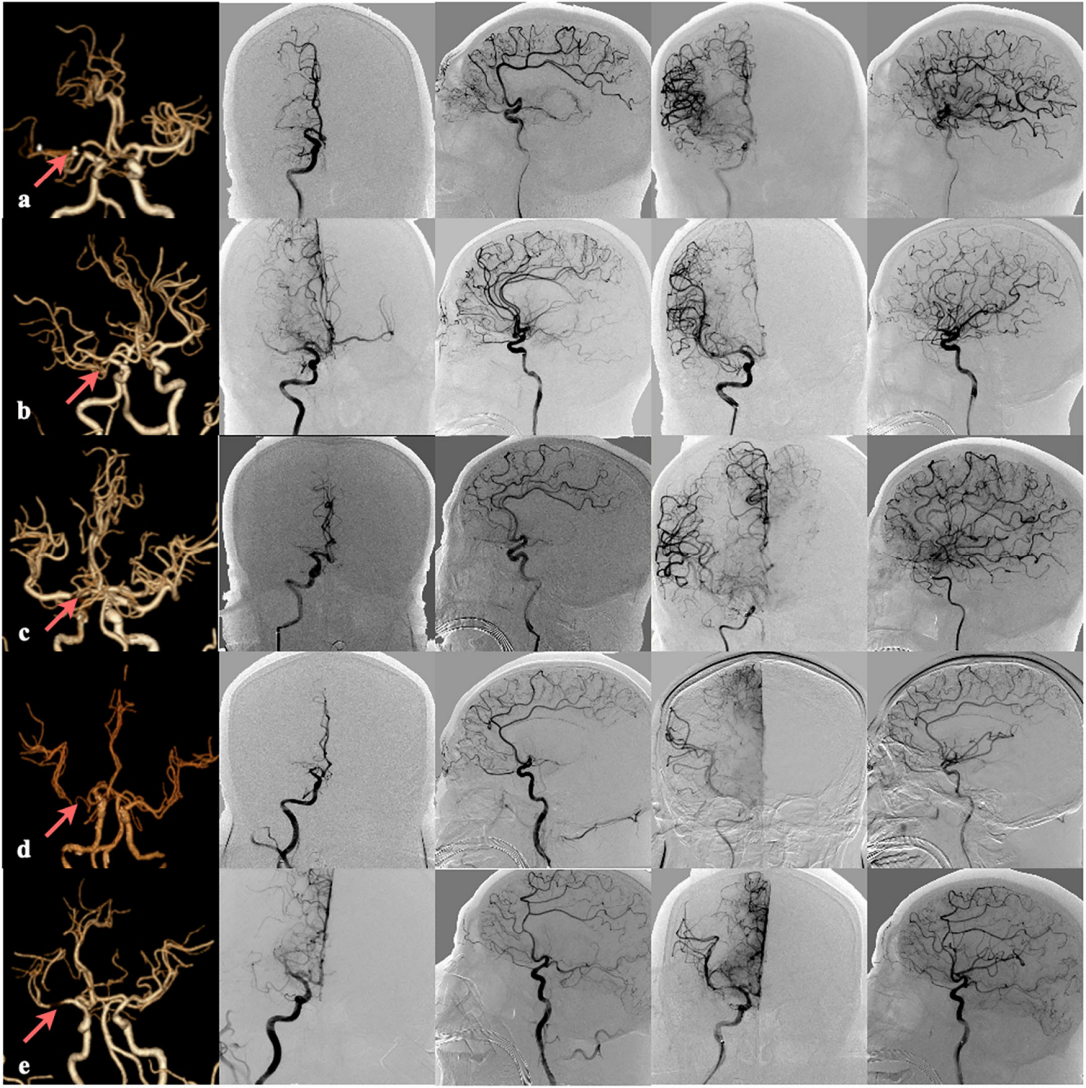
Representative cases of acute ischemic stroke with right-sided large-vessel occlusion treated by EVT in peri-clot model: a Proximal M1 occlusion with predicted and confirmed complete reperfusion with good clinical outcome (90-day mRS 0); b M1 occlusion with predicted and confirmed complete reperfusion with good clinical outcome (90-day mRS 1); c Terminal internal carotid artery occlusion with predicted non-complete but ultimately confirmed complete reperfusion with good clinical outcome (90-day mRS 1); d M1 segment terminus occlusion with predicted complete but ultimately non-complete reperfusion with poor clinical outcome (90-day mRS 4); and (e) M1-M2 junction occlusion with predicted and confirmed non-complete reperfusion with poor clinical outcome (90-day mRS 3). The first column shows CTA images with red arrows indicating the occlusion site; the second and third columns display pre-thrombectomy DSA (anteroposterior and lateral views); and the fourth and fifth columns demonstrate post-thrombectomy DSA (same projections) with corresponding reperfusion outcomes. EVT, endovascular therapy; CTA, computed tomography angiography; DSA, digital subtraction angiography
